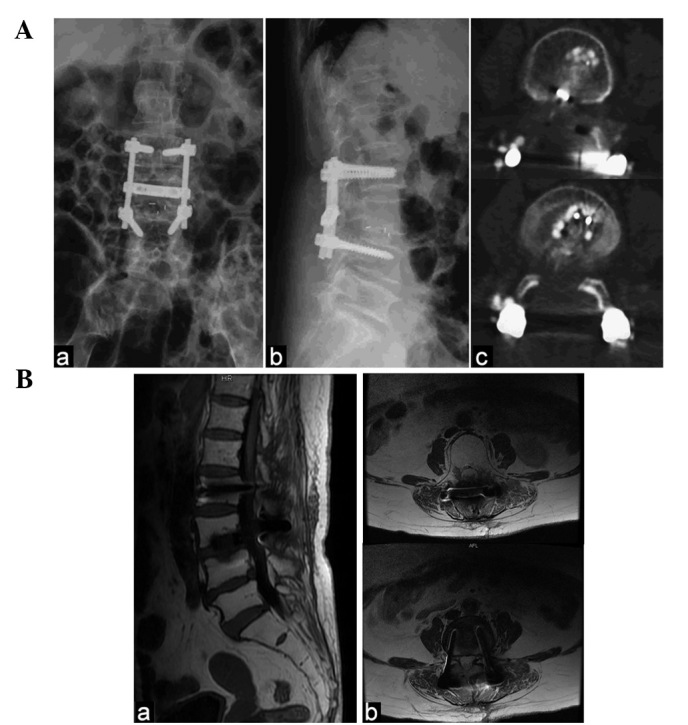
(A) Post-surgery (a and b) lumbar radiograph and CT scan. (c) Removal of the tumor, L3 lamina and inferior articular process and spine fixation at the L2–4 level and interbody fusion at the L3–4 level. (B) At 13 months post-surgery, (a and b) the MRI showed no evidence of recurrence. Aa, posteroanterior; Ab, lateral; Ac, transverse plane; Ba, T1/sagittal plane; Bb, T1/transverse plane. CT, computed tomography; MRI, magnetic resonance imaging.
